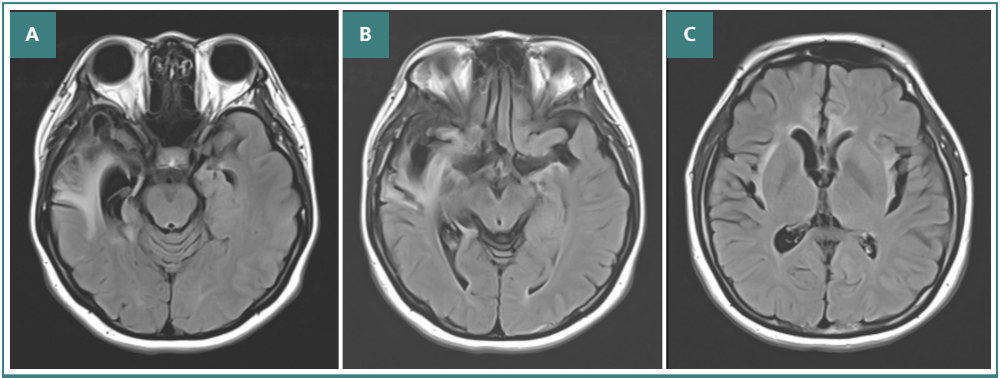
Second Brain MRI (FLAIR) after 6 months showed cerebromalacia in the right anterior temporal region combined with a dilated right ventricle horn (A-C).
